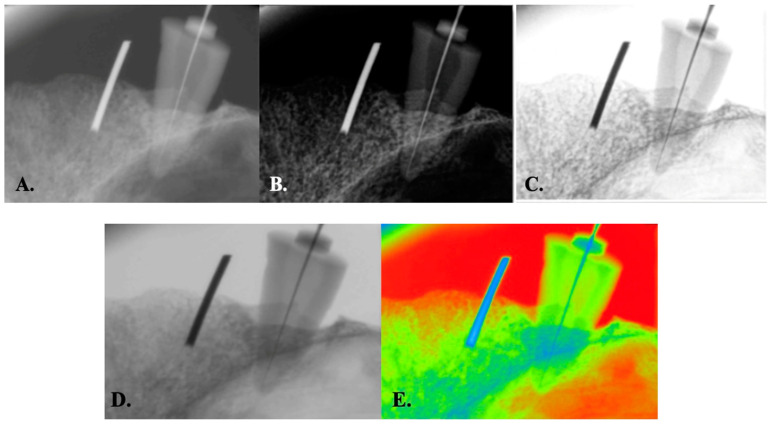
The radiographic images were obtained via five image modules: original (A), autoenhancement (B), autoenhancement+negative (C), original+negative (D), and colored (E) modules.
